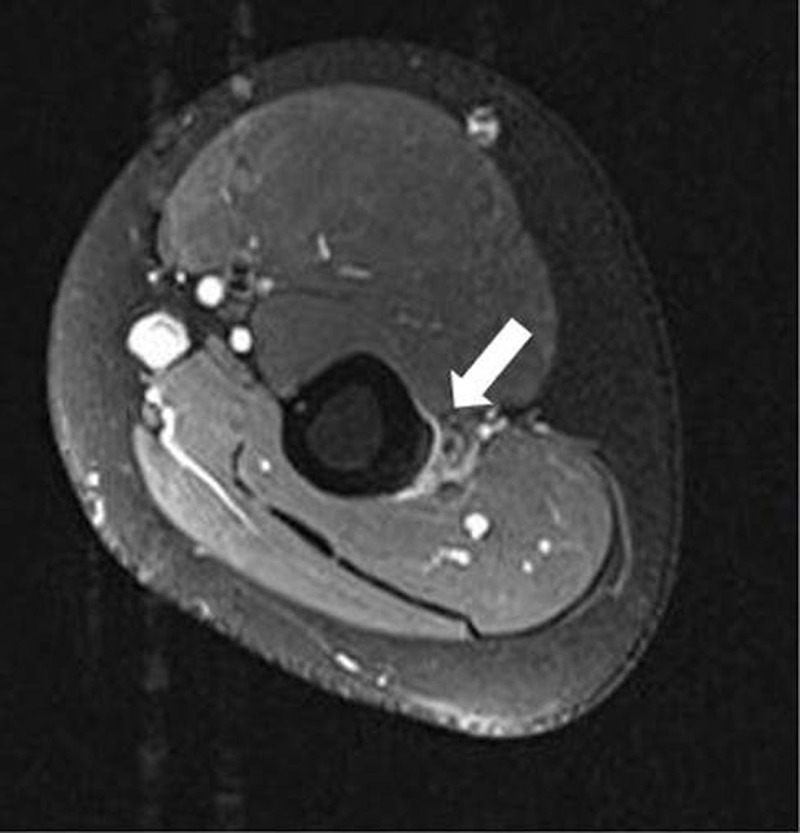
Subsequent computed tomography (CT) excluded an osteoid osteoma and confirmed focal cortical thickening with solid periosteal reaction (*Figure 3*, arrow).
